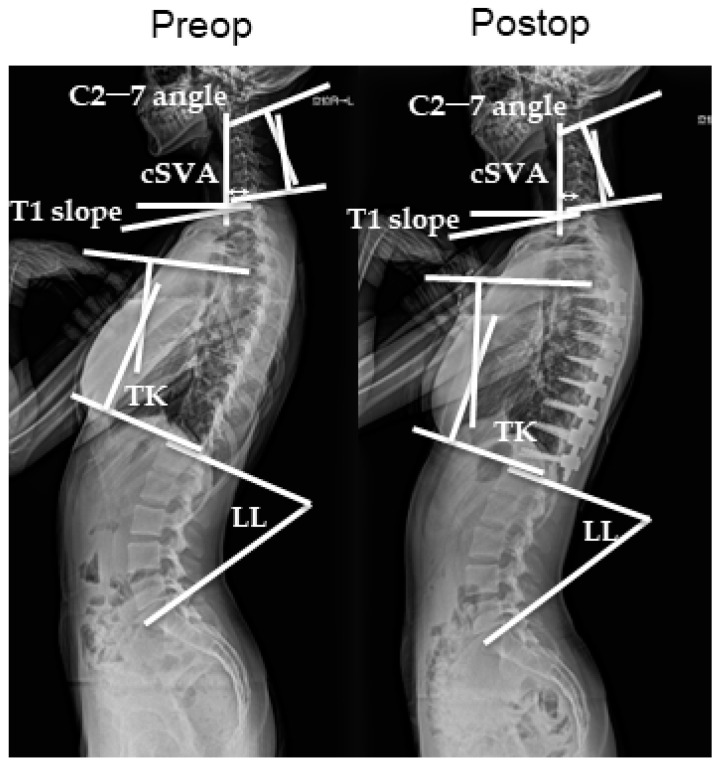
Standing lateral X-ray showing the calculation scheme before and after surgery.
